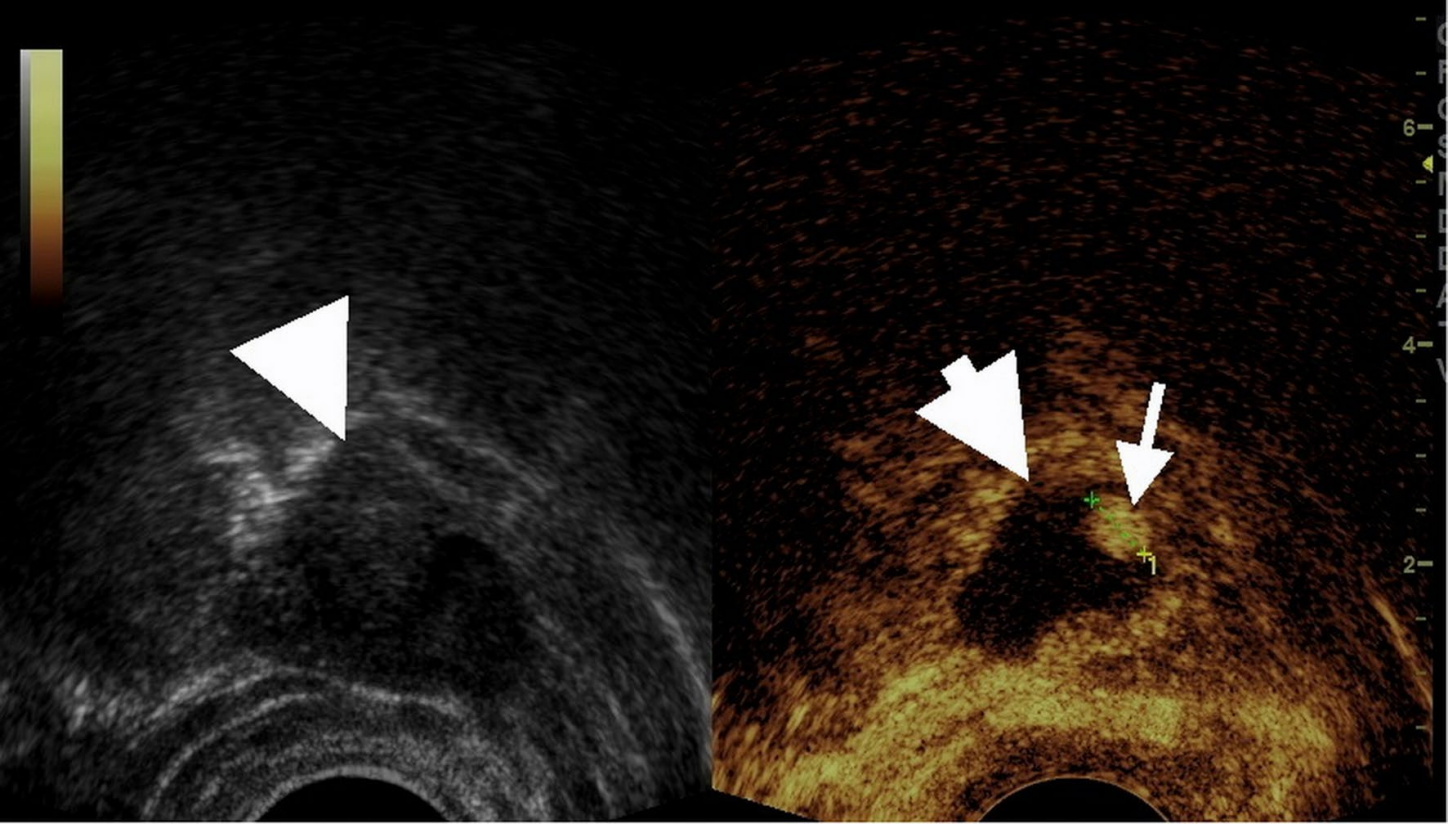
Transvaginal contrast enhanced ultrasound (CEUS) showed a adnexal mass of unknown origin. The patient was a 23-year-old woman who presented with amenorrhea and vaginal bleeding. The patient’s blood HCG level was 1010 mIU/mL, and the time interval between CEUS and blood testing was less than 6 h. A mixed echoic mass measuring 2.4 cm×2.1 cm was seen beside the right ovary (left image). CEUS showed heterogeneous enhancement of the mass. The thick arrow indicated the enhanced cyst wall and the thin arrow indicated the enhanced villous tissue (right image). The ultrasound diagnosis was ectopic pregnancy with the location to be undetermined and the possibility of ovarian pregnancy was relatively high. The final surgical diagnosis confirmed it as a right ovarian pregnancy, with the villous tissue measuring 0.8 cm×0.8 cm, similar to the ultrasound measurement (0.8 cm×0.6 cm). The non-enhanced areas within the lesions were surgically confirmed to be blood clots
